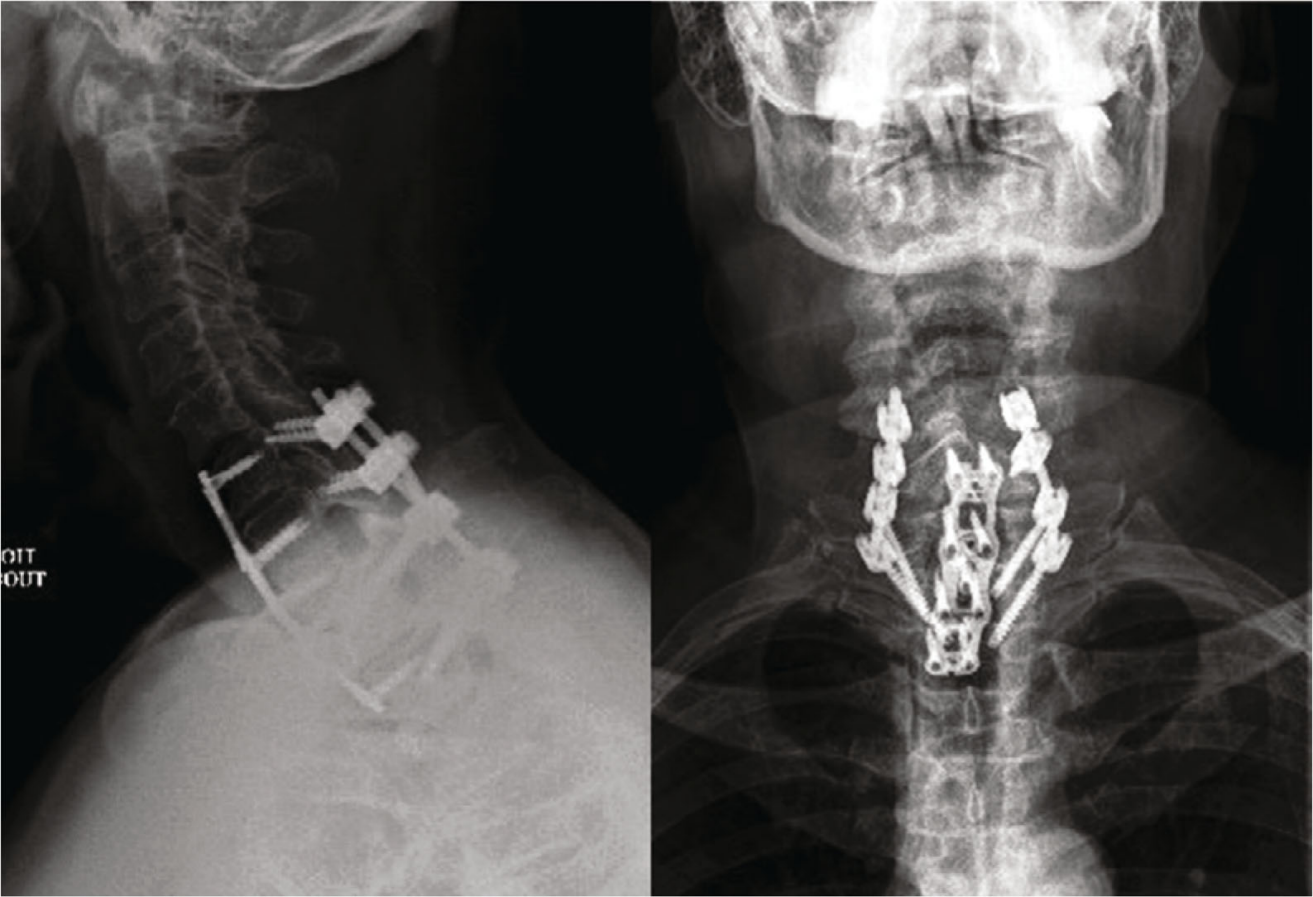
X-ray at one-year follow-up shows a well bony fusion.
